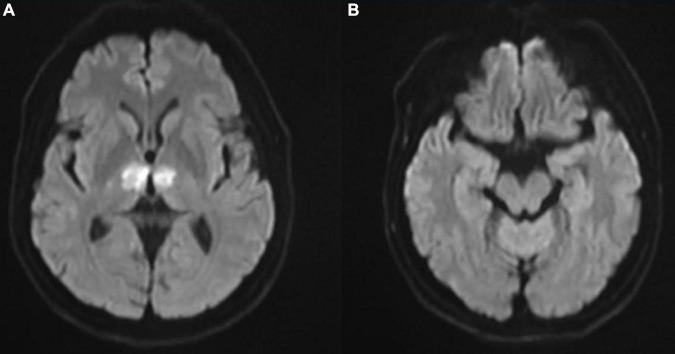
(A) Axial diffusion-weighted magnetic resonance imaging (MRI) showing bilateral paramedian thalamic hyperintense signal due to restricted diffusion and (B) no involvement on the midbrain level.
